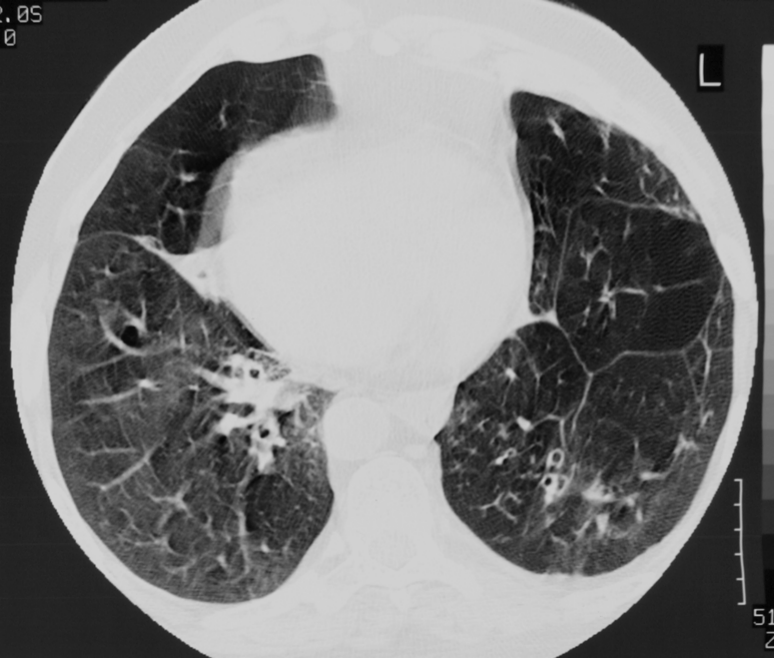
CT scan of the thorax showing dextrocardia and bronchiectasis of the lungs.
